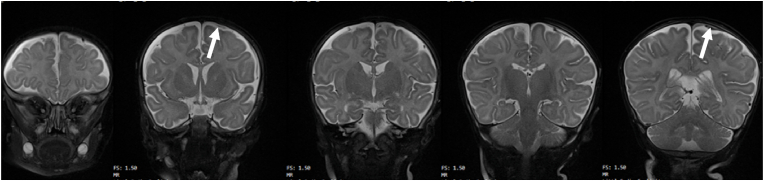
Case #1. Series of coronal MRI scans showing very thin subdural fluid layers (arrows) compatible with chronic SDH (hygroma).
